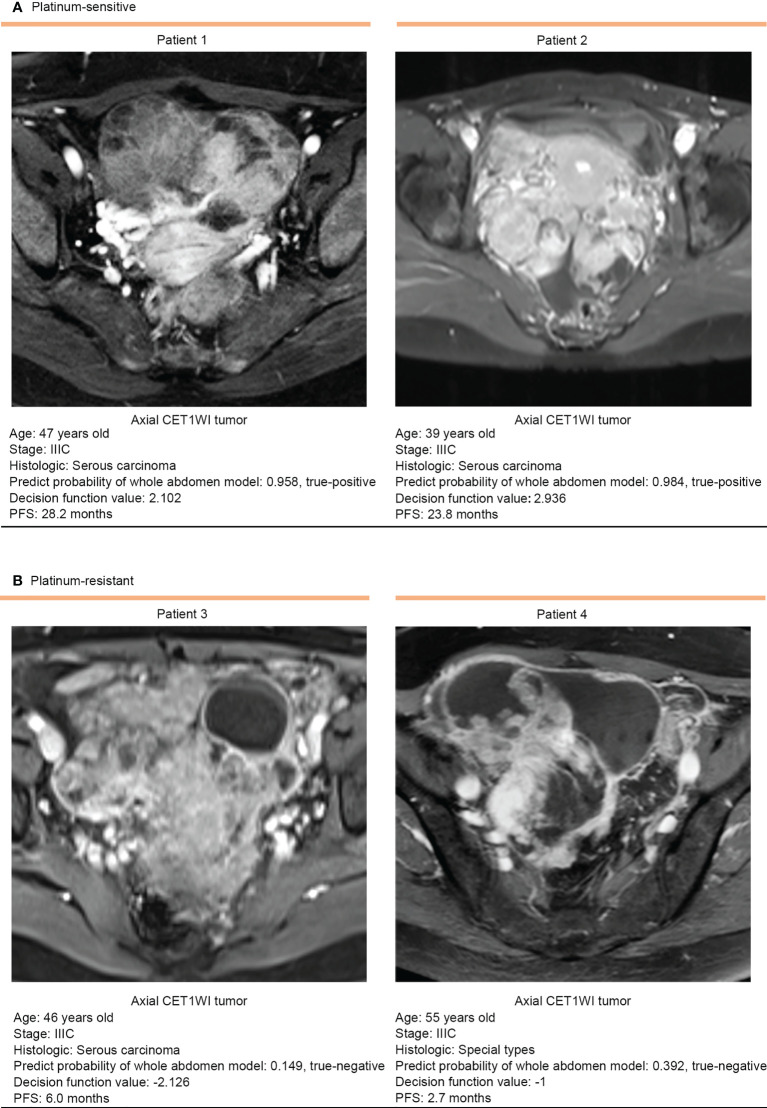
Representative prediction results of the whole abdomen model. (A) Patients 1 and 2 were platinum-sensitive and (B) patients 3 and 4 were platinum-resistant. The platinum sensitivity of patients was accurately assessed according to the decision function value by the cutoff value of 0.00. CE-T1WI, contrast-enhanced T1-weighted imaging.
